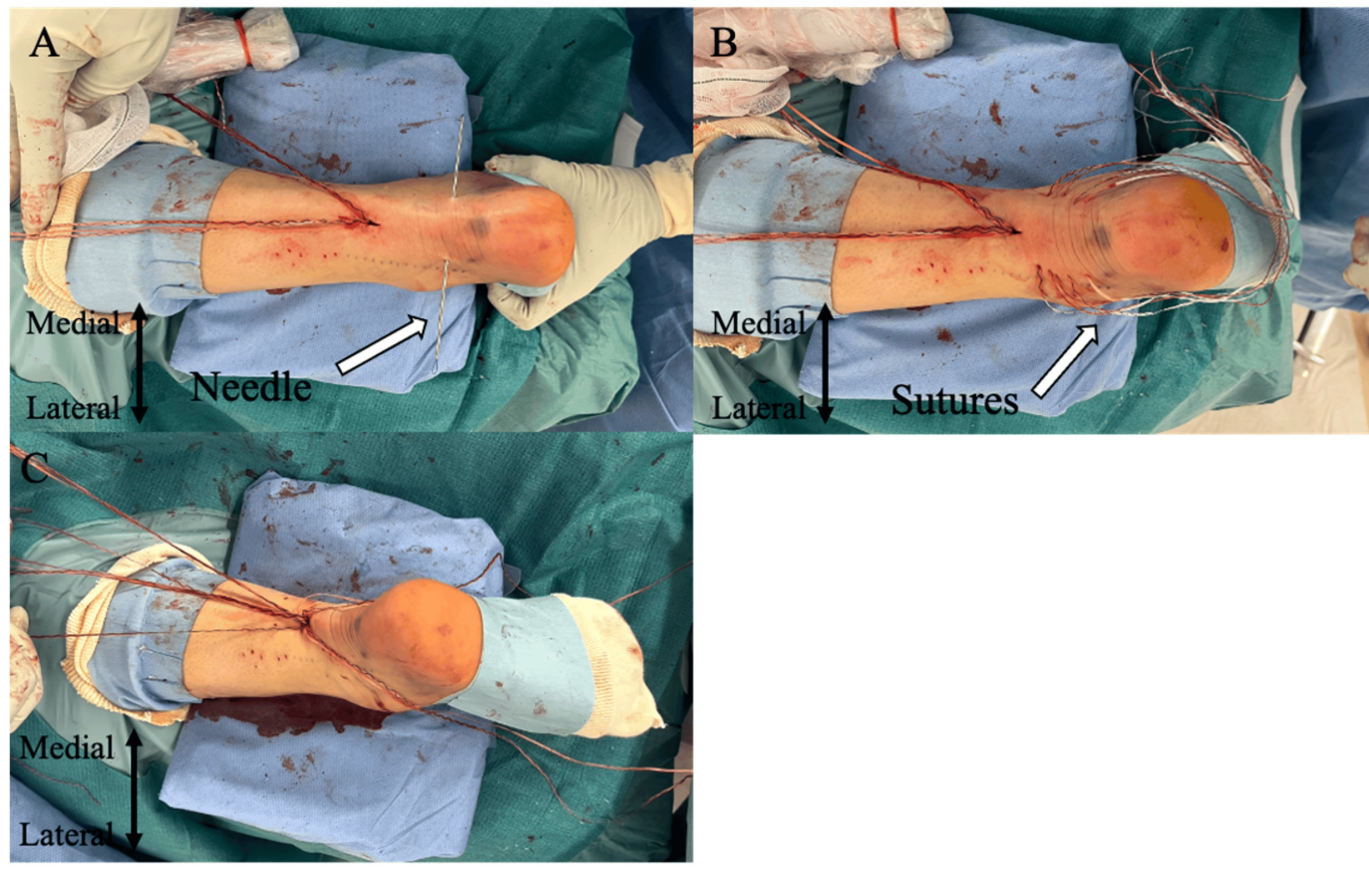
Suture passage and locking suture at the distal Achilles tendon stump under ultrasonographic guidance, replicating the proximal stump technique A: Needle insertion at the distal stump; B: Completion of suture passage; C: Completion of suture retrieval and locking suture Image source: Authors
